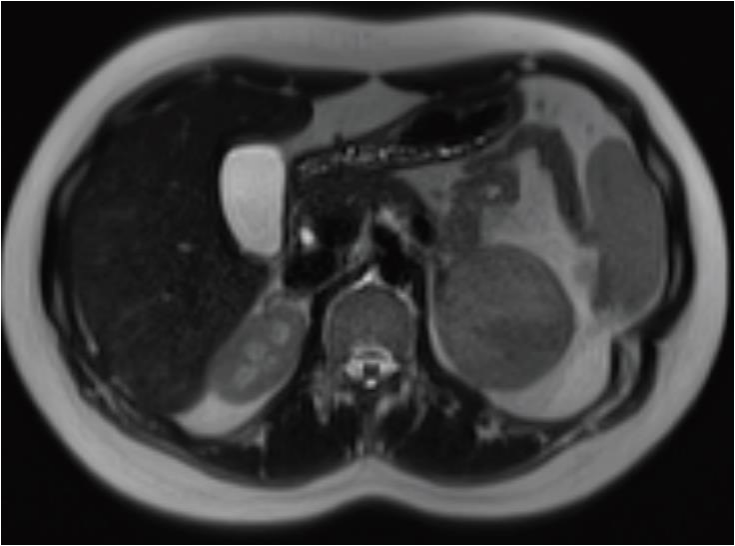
Abdominal CT scan demonstrating the androgen-producing adrenal tumor of the left adrenal gland in the patient of case 4.
